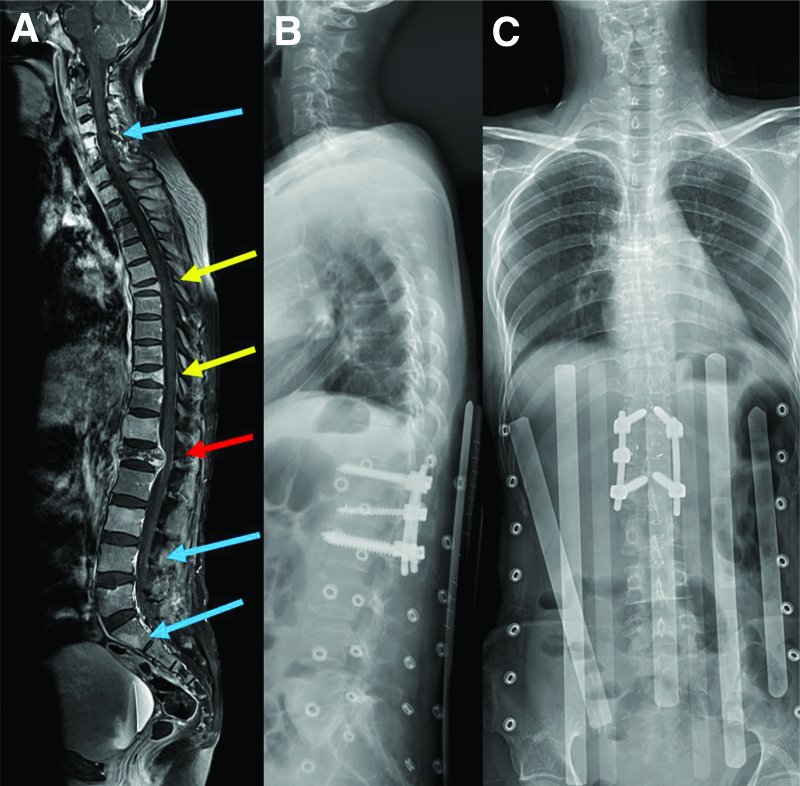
A 37-year-old woman diagnosed with parotid gland cancer presented to the hospital with sudden leg weakness and severe back pain. Preoperative sagittal MRI (A) reveals several vertebral metastases, including at the C4–6, L4, and S1 vertebral body masses (indicated by blue arrows); T6 and T10 pathologic compression fractures (indicated by yellow arrows); and L1 pathologic compression fracture (indicated by a red arrow). The presence of adjacent metastatic lesions made it difficult to perform the traditional long level surgery, therefore, we performed TLSF at the offending L1 level. Postoperative lateral (B) and anteroposterior (C) X-ray films show well-fixed screws at T12 to L2. MRI = magnetic resonance image, TLSF = thoracolumbar short fixation.
